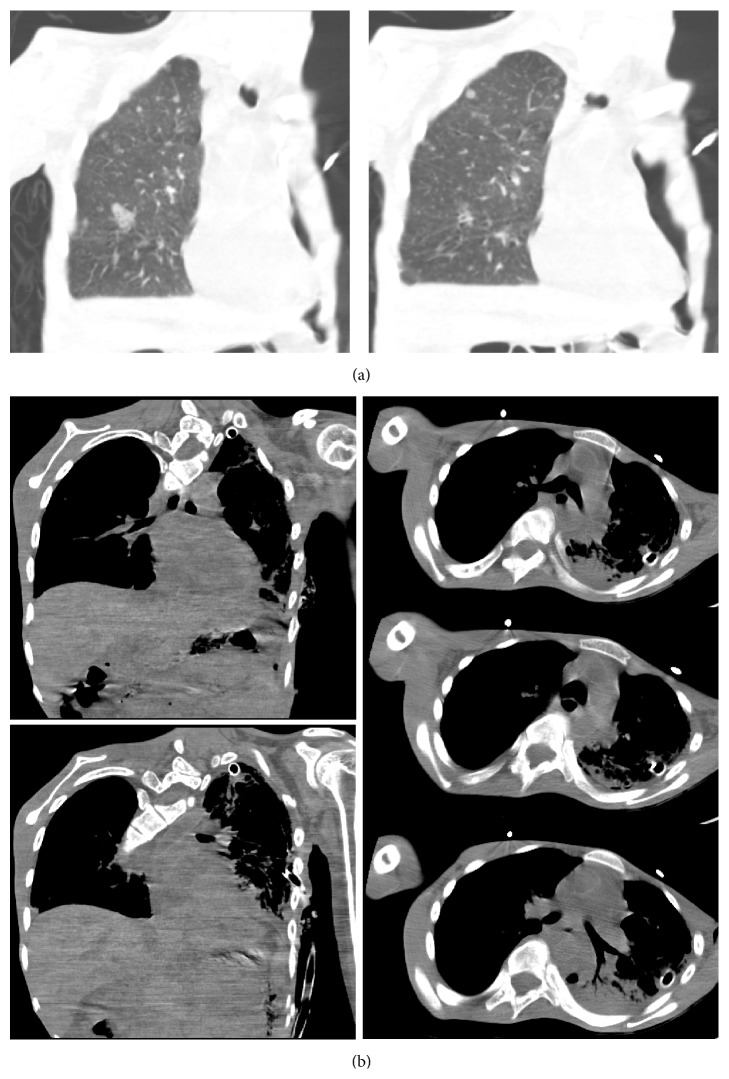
(a) Chest CT different cuts from lung showing right side multiple small nodules. (b) Mediastinal window showing no signs of air in the mediastinum.
